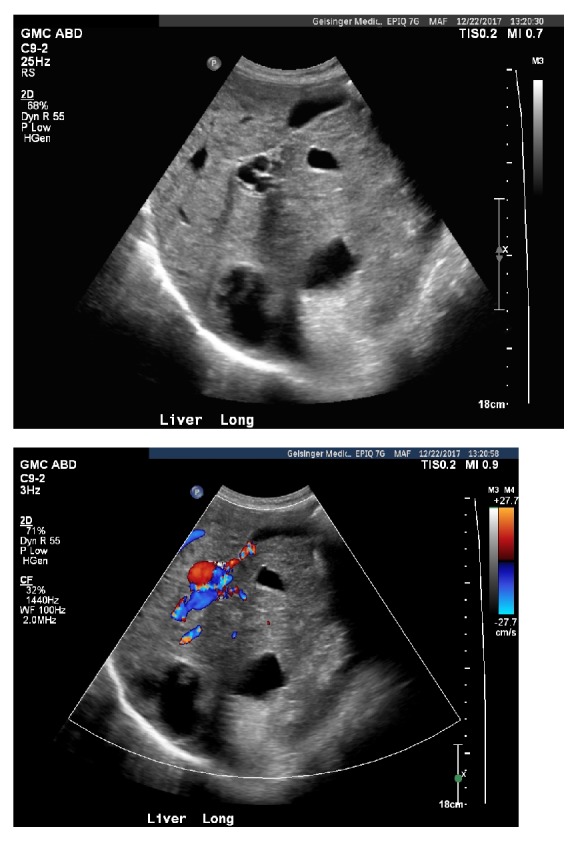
(a) Ultrasound gray scale image shows multiple anechoic structures in the liver with surrounding echogenicity. There is flow on color Doppler (b), consistent with hepatic arteriovenous malformations.
